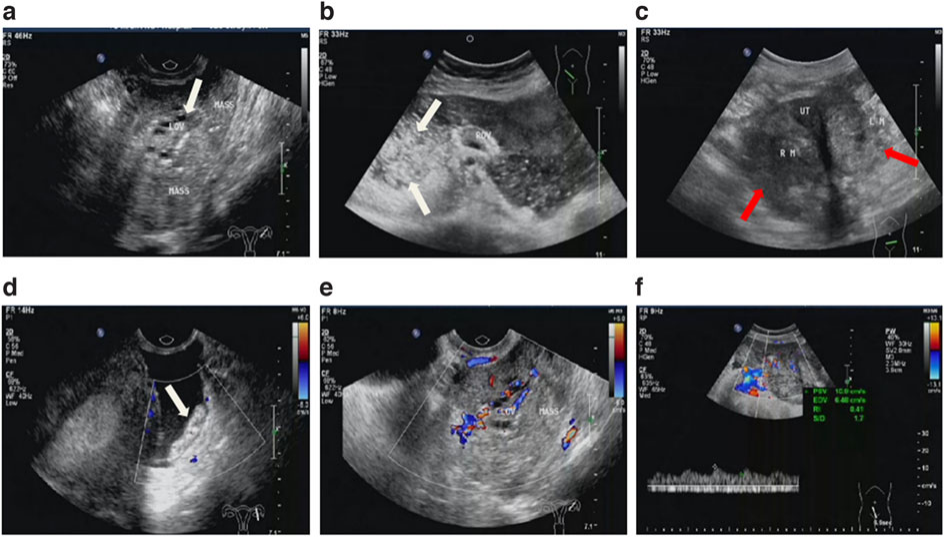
Conventional ultrasonic characteristics of OSSPBT. (a) A large area of solid echo was seen around the ovary, and a normal ovary with small follicles (white arrow) was seen in the mass. (b) The ovary is surrounded by a large number of solid mixed echoes, and a large number of speckled strong echoes are seen, showing “blizzard” sign (white arrow). (c) MOSC was bilateral ovarian irregular solid or mixed echo mass (red arrow), no normal ovarian tissue echo. (d) There were tumor planting nodules (white arrow) and punctate blood flow signals in the retroperitoneum of the pelvic cavity. (e, f) CDFI showed mild or moderate blood flow signals in the tumor, PSV: 10.9 cm/s, RI: 0.41, and color score: 3. CDFI, color doppler flow imaging; LM, left ovarian lesion; Mass, lesions; MOSC, malignant ovarian serous cystadenocarcinoma; OV, ovary; PSV, peak systolic velocity; RI, resistance index; RM, right ovarian lesion; UT, uterus.
